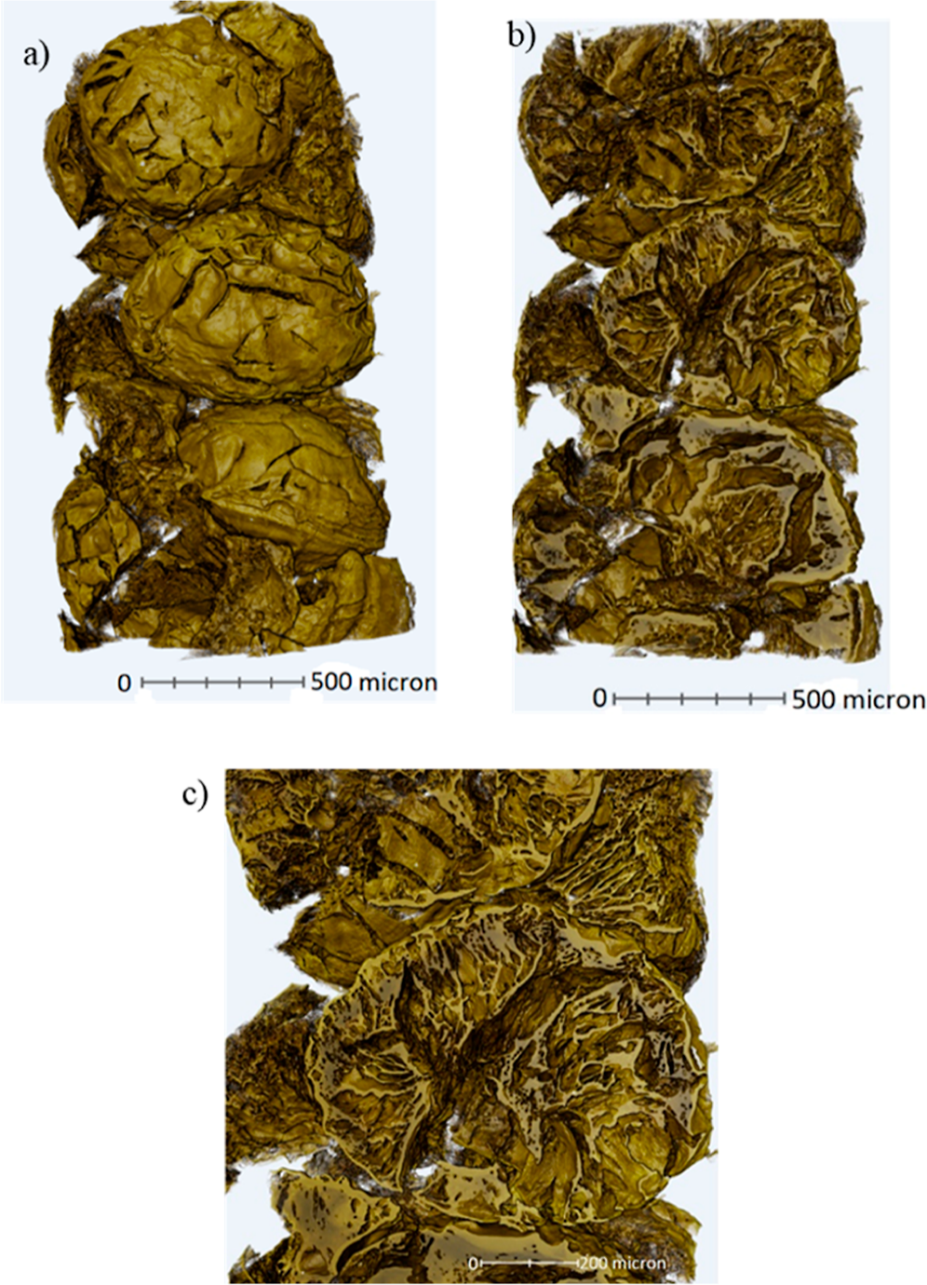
3D rendered CT scan images of 0.6 mm beads (a) outer surface, (b)
